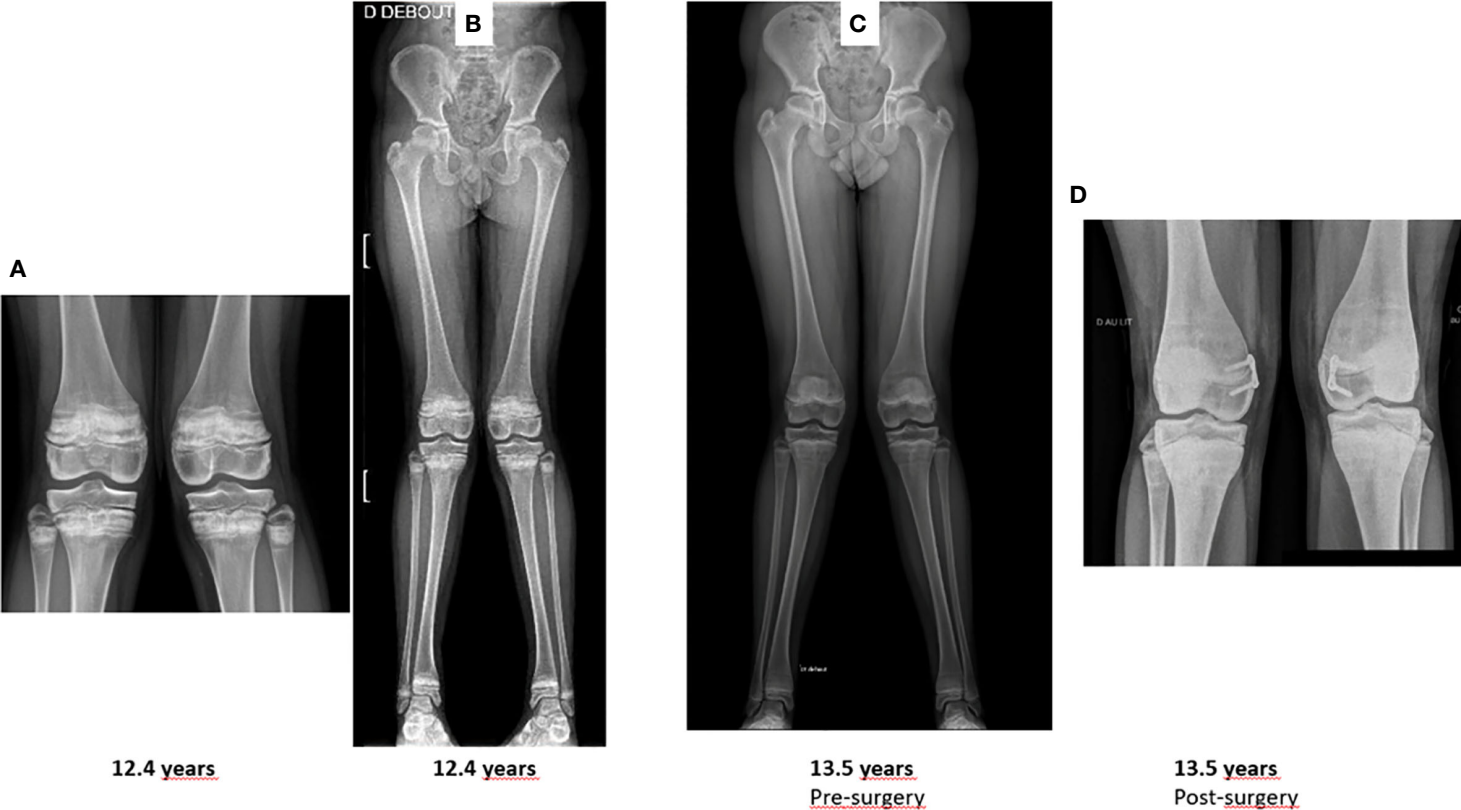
Knees and lower limb radiographs. Lower limbs were imaged standing using EOS. (A, B) lower limb deformities at the age of 12.4 years. (C) deformities worsened after one additional year, (D) post-surgery radiographs of knees.
